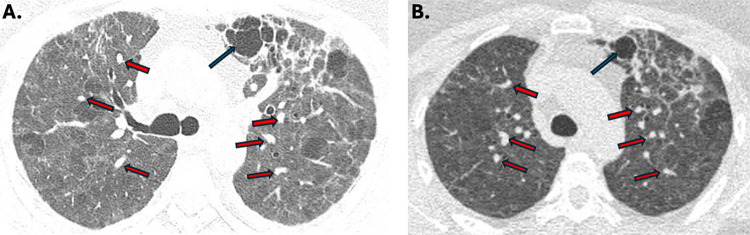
Thorax CT: cavitary lesion (blue arrow) and numerous nodules in lungs. Eighty-eight per cent lung involvement, thickened bronchial walls in the lower lobes, multiple ground-glass opacities, well circumscribed nodular consolidations in the lower lobes, cavitary lesions and areas of emphysema.
